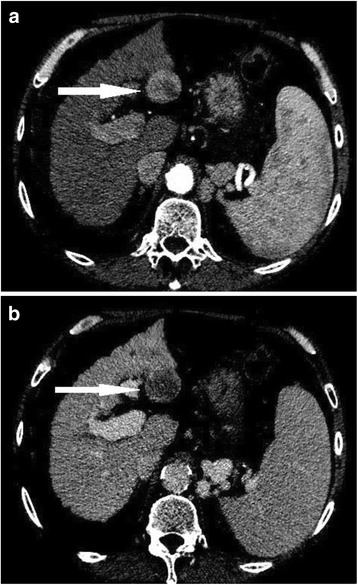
54 year old male with hepatitis C cirrhosis. CT shows an arterial enhancing nodule a with washout of contrast in the delayed phase b consistent with hepatocellular carcinoma
